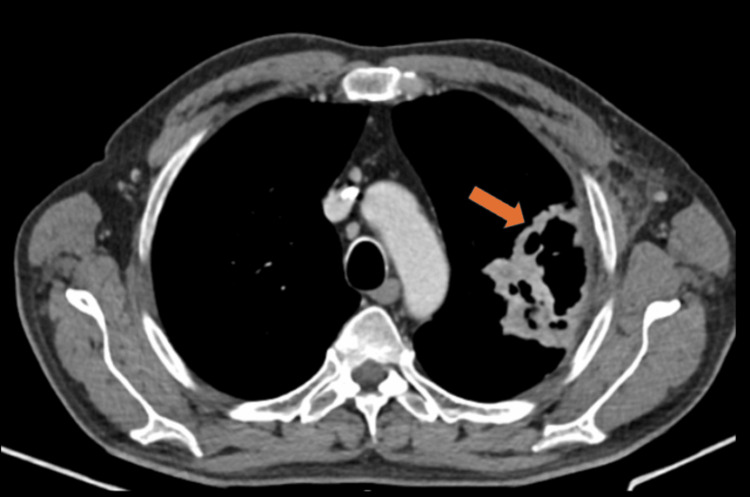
CT scan showing the dimensional increase of the lung lesion, indicating disease progression CT: computed tomography
